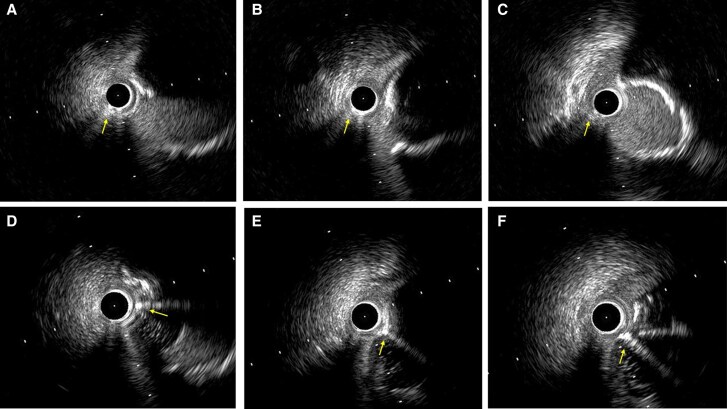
(*A–C*) Intravascular ultrasound images obtained sequentially from proximal to distal segments within the proximal left anterior descending, showing migration of both the intravascular ultrasound catheter and an additional wire into the subintimal space. (*D–F*) Corresponding intravascular ultrasound images following balloon inflation demonstrating repositioning of a semi-compliant 2.0 mm balloon and an additional wire from the subintimal space into the true lumen. The arrows indicate the additional wire.
